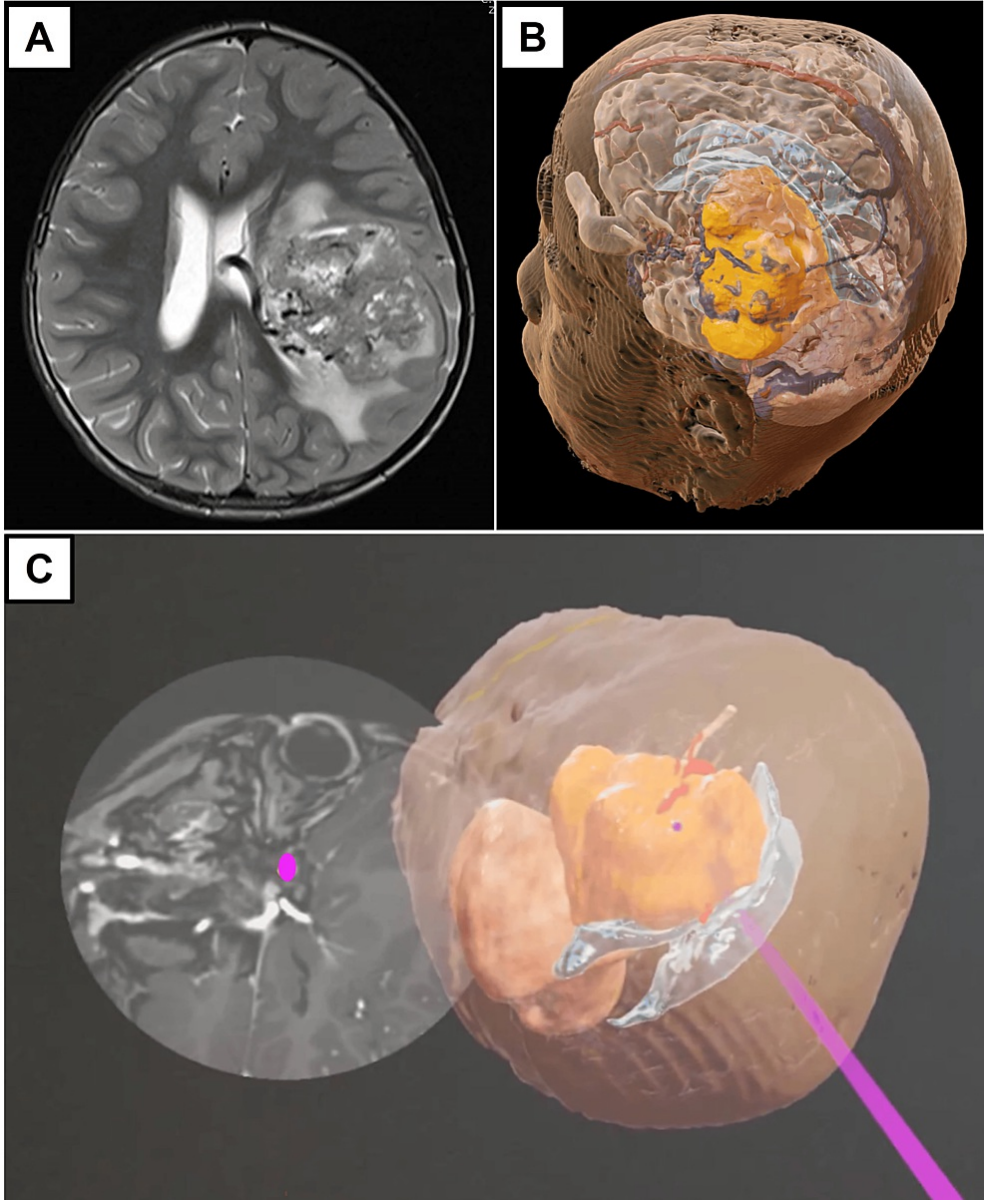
Summary of images used for Case 2. (A) Representative preoperative magnetic resonance imaging (MRI) image in the T2-weighted sequence depicting a highly vascular giant supratentorial brain tumor (GSBT). (B) Representative image of the three-dimensional (3D) model after segmentation of the tumor (yellow-gold) and adjacent ventricle and parenchyma. (C) Representative streamed augmented reality (AR) images placed next to the corresponding MRI brain slide to allow the neurosurgical team to know the location of the feeding vessels (red) in relation to the tumor (magenta).
